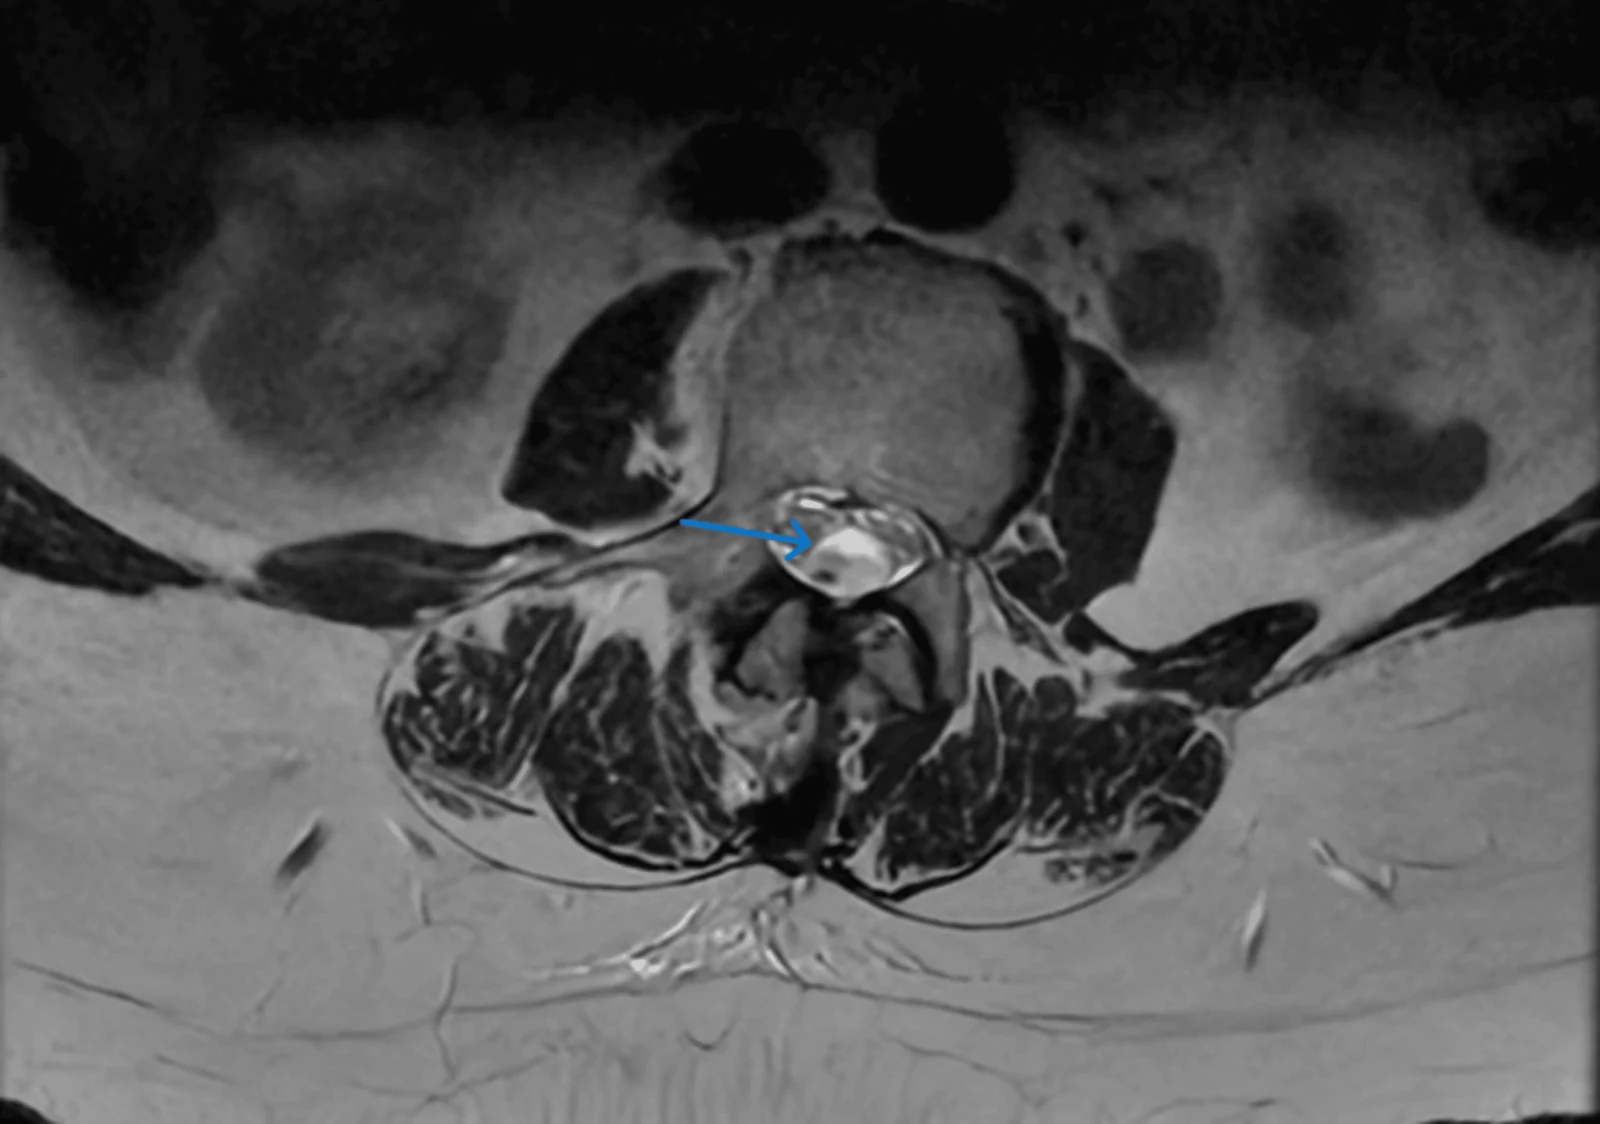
Axial T2‑weighted MRI at L3 Axial T2‑weighted MRI at L3 showing ventral displacement of the cauda equina caused by the dorsal collection (arrow).
